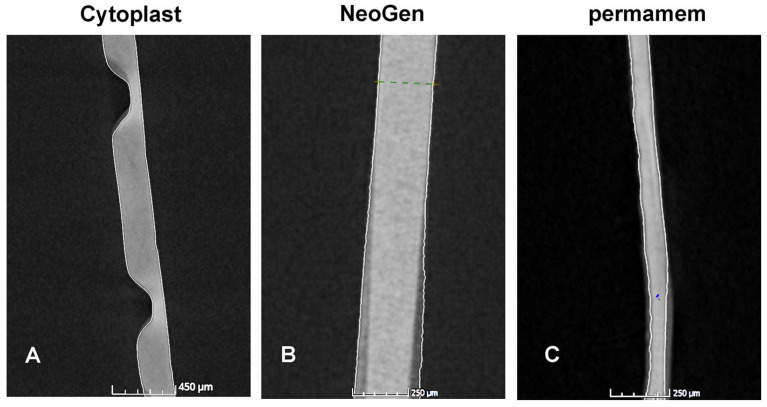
Nano-CT examination of the tested membranes. Representative axial sections from textured and non-textured membranes. (A) Cytoplast^TM^ TXT-200 textured membrane with hexagonal shaped indentations on the soft-tissue site and flap surface in the bone tissue site; (B) NeoGen^®^: the thickest non-textured membrane; (C) permamem^®^: the thinnest non-textured membrane.
